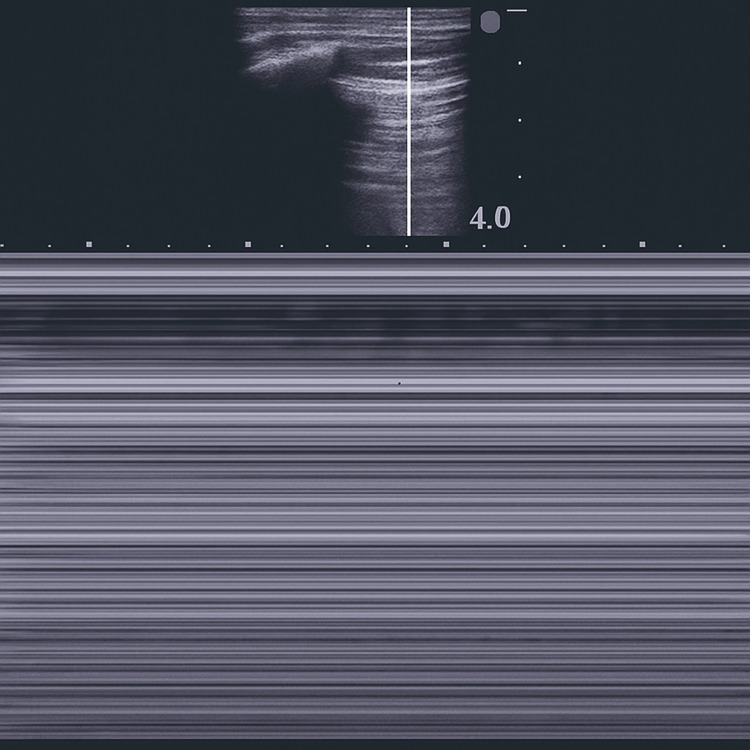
M-mode ultrasound demonstrating the barcode sign in pneumothorax M-mode lung ultrasound image showing the barcode sign (also known as the stratosphere sign), characterized by a pattern of horizontal lines throughout the image, without the granular appearance seen in normal lung sliding. This pattern reflects absent pleural sliding, which is highly suggestive of pneumothorax at the examined site.
